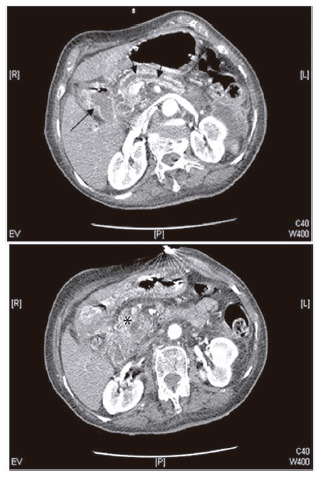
Abdominal computed tomography shows a heterogeneous mass of the head of the pancreas (*), which leads to marked dilatation of the Wirsung duct and duodenum (arrows)
